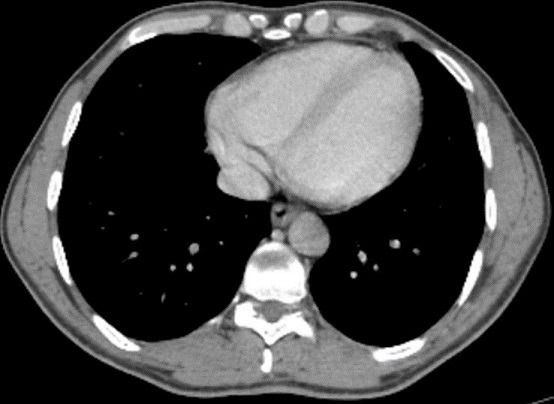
Chest CT scan showing disappearance of the rib metastasis
